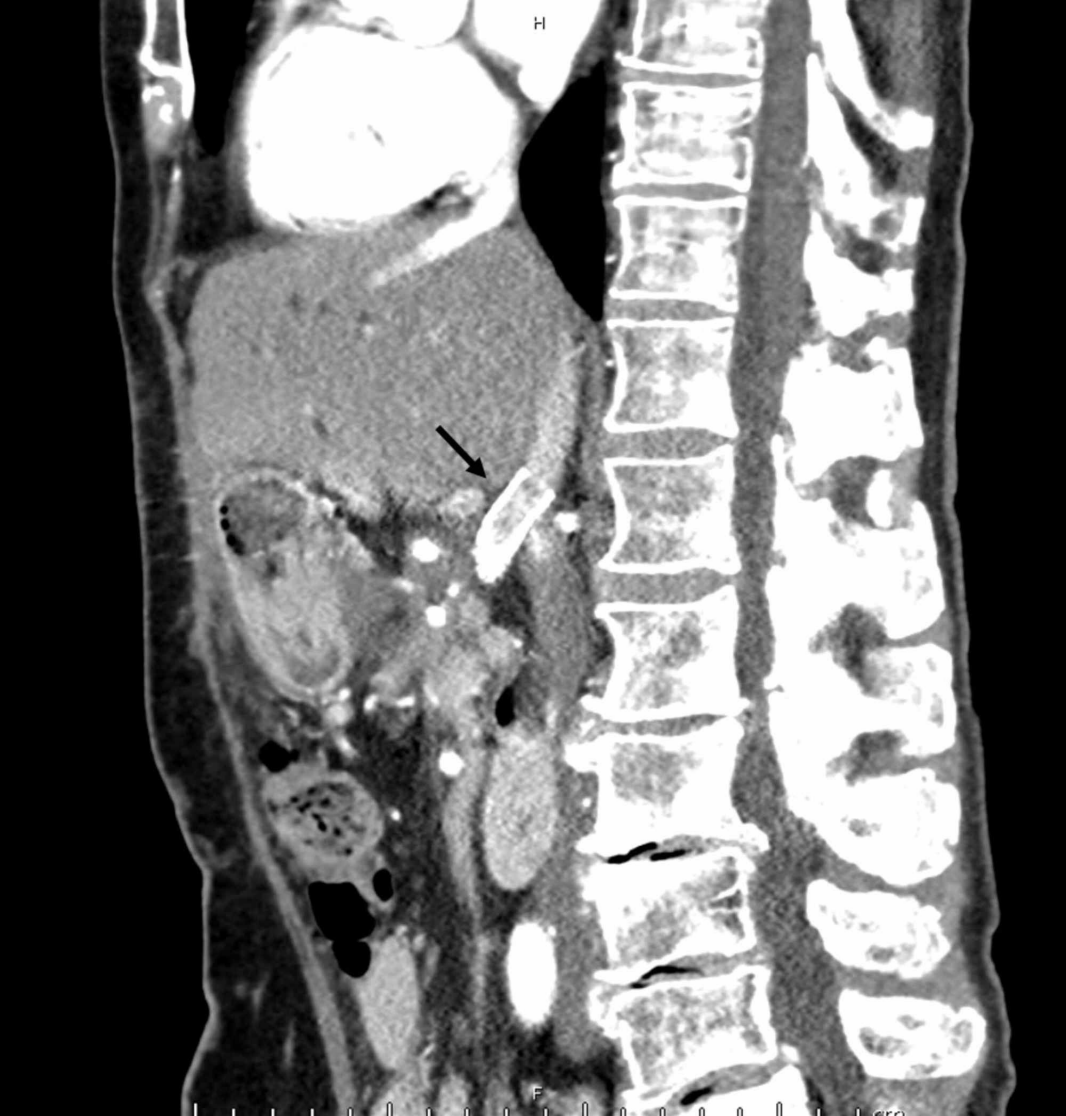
Computed tomography of the abdomen with contrast (sagittal view) shows patent shunt with stent (arrow) between superior mesenteric vein and inferior vena cava
